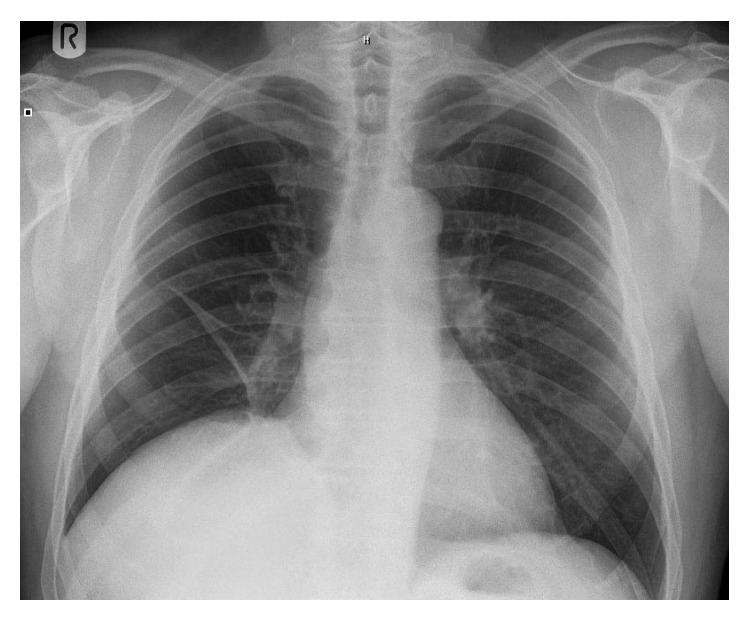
Preoperative chest radiograph in a 64-year-old man with right-sided stenosis at C3/4 and C4/5 demonstrating an elevated right hemidiaphragm.
